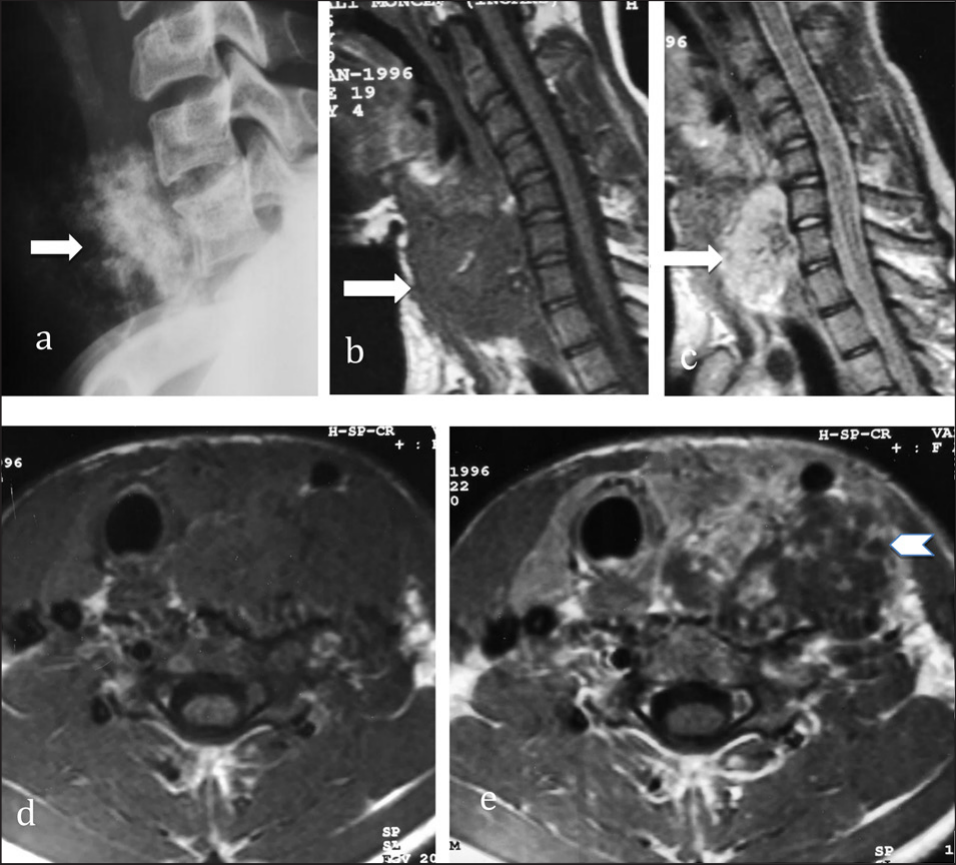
Chondrosarcoma of C7. (a) Lateral radiograph of cervical spine shows a large iuxta-osseous calcified mass arising from the vertebral body of C7 (arrow). (b, c, d, e) MR: Sagittal T1-WI, Sagittal T2-WI, Transversal T1-WI, Transversal T1-WI after gadolinium contrast injection show lobulated mass of intermediate signal intensity on T1-WI and high signal intensity on T2-WI (arrows) with ring-and-arc enhancement (arrowhead).
